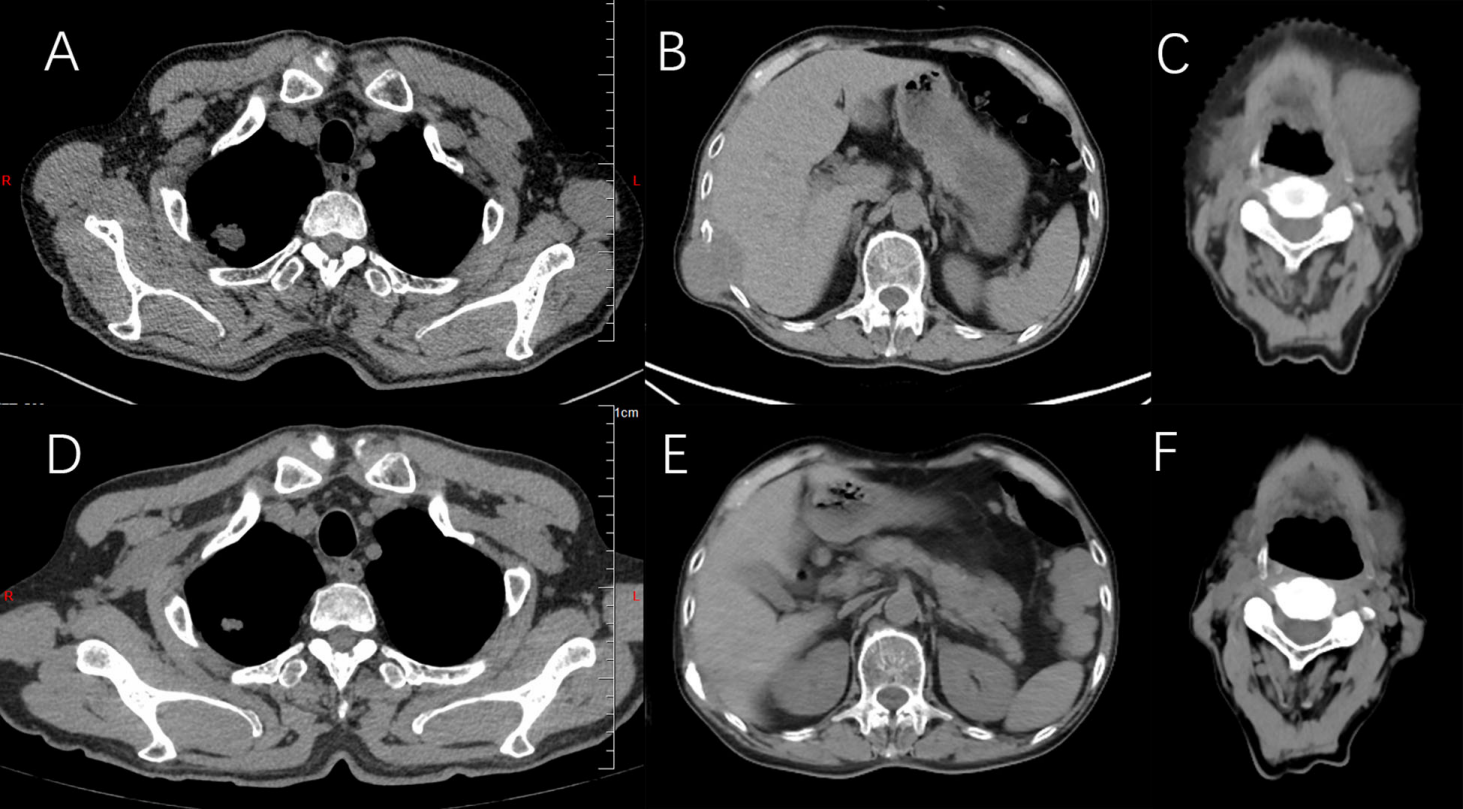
Computed tomography. (A) Obvious occupying lesion in the right upper lung lobe. (B) Metastasis occurred in the right lower chest wall and was evaluated as PD. (C) Metastasis occurred in the neck and was evaluated as PD. (D) After the PP chemotherapy regimen, the lesion decreased in size. (E) Radiotherapy was administered to a mass in the right lower chest wall, resulting in a significant reduction in size. (F) The neck mass was treated with radiotherapy, and the efficacy was evaluated as PR.
